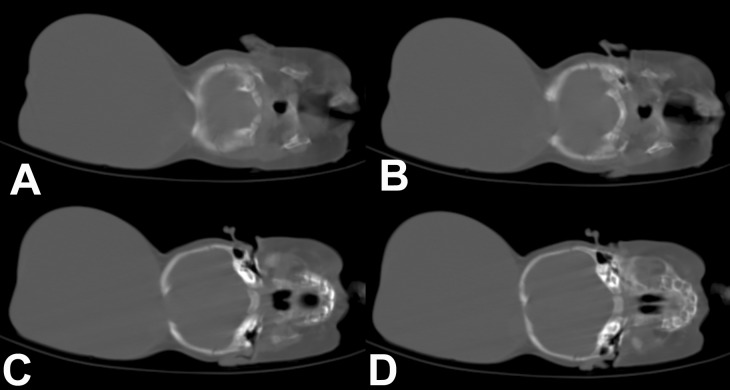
Figure 3: CT scan brain (bone window) showing large defect in the occipital bone with sclerosed margins.
